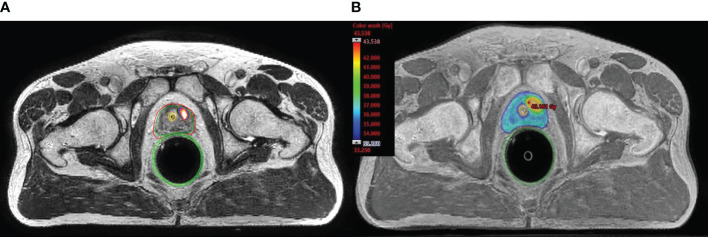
Typical planning fused image set (MRI and PSMA-PET/CT) and dosimetric results. (A) shows MRI/PET-CT fusion with identification of intraprostatic tracer-avid recurrence. (B) shows color-wash dose distributions representative of the typical plan with the boost dose (40 Gy) covering the dominant lesion.
